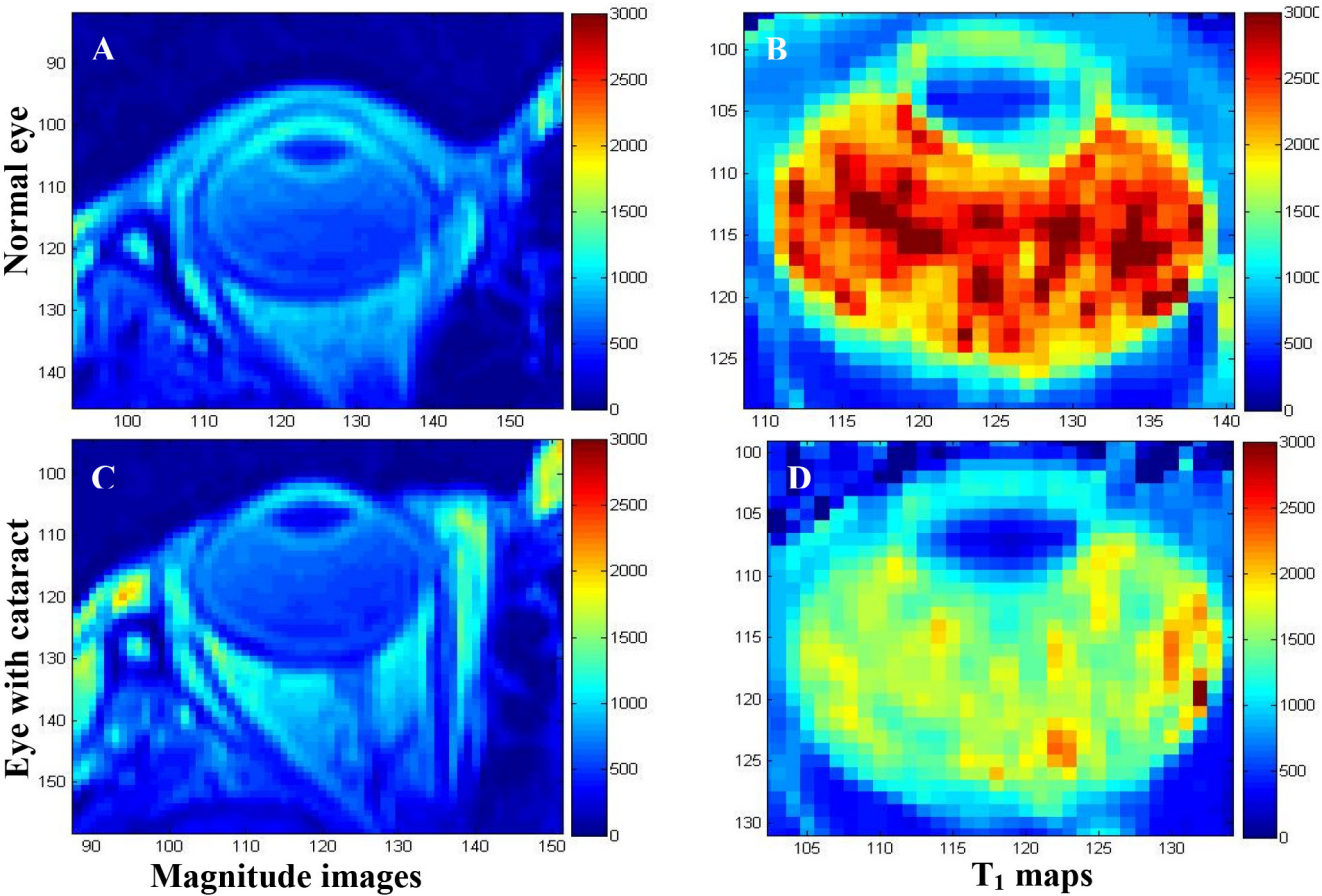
T_2_-weighted magnetic resonance images: A, C and the corresponding T_1_ maps: B, D: The image and T_1_ map in panels A and B are that of a normal eye, while those in panels C and D are of an eye with cataract. No significant differences were identified between the magnitude images of the normal eye in A and that of the eye with cataract in C. The T1 values calculated in ms on the T1 maps show that the T1 values of the eye with cataract in D were two times lower than that of the normal eye in B in the vitreous and aqueous humors. The spatial resolution of the images is 780×1560×2000 μm^3^, and the images were acquired in approximately 1 min. The images were reproduced from [33] with permission from the Romanian Society of Medical Imaging.
